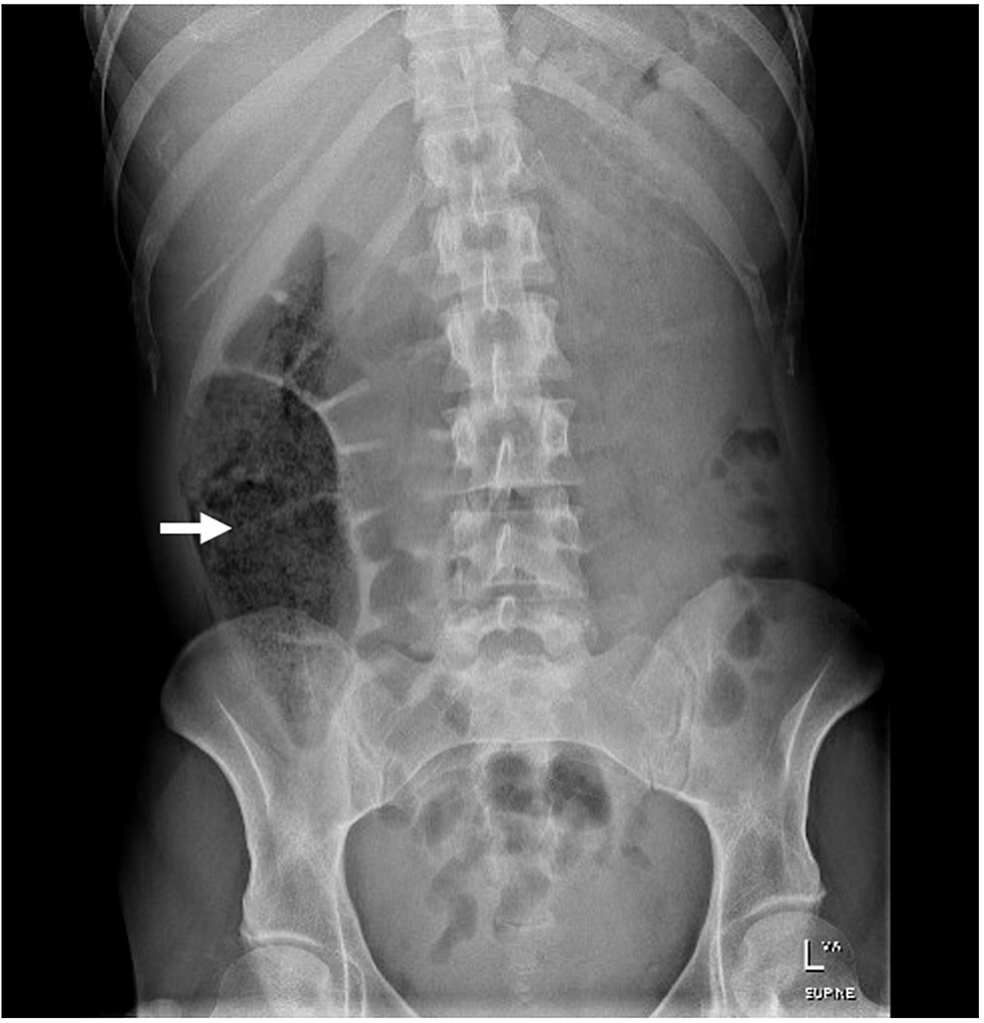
Abdominal X-ray showed progression of the trichobezoar into the caecum and ascending colon
